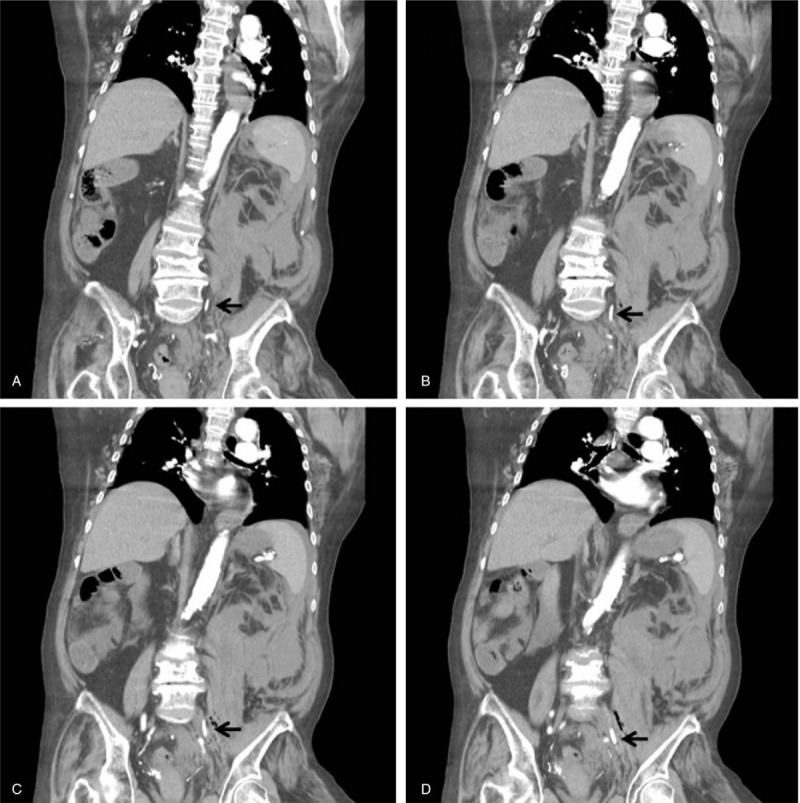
Computed tomography image of the patient showing a double-lumen catheter tip protruding from the left iliac vein (arrow) and hematoma in the retroperitoneal space and pelvic cavity.
